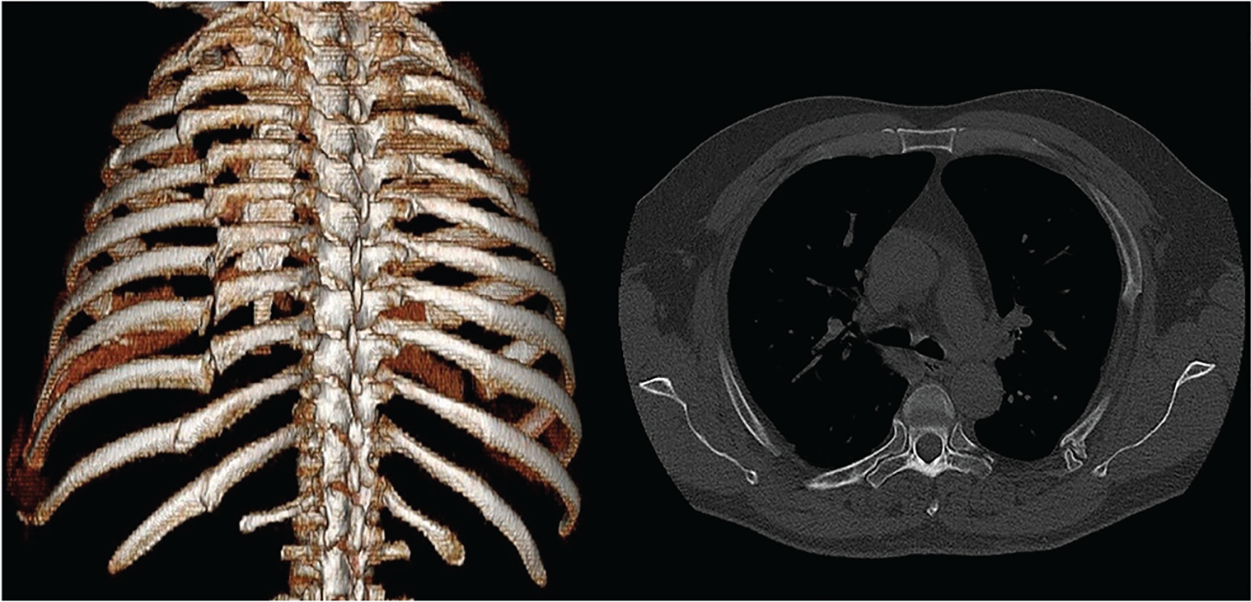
Computed tomography demonstrating multiple healed posterior rib fractures of ribs 2 through 12 with chronic rib fracture nonunion of ribs 6 through 10
